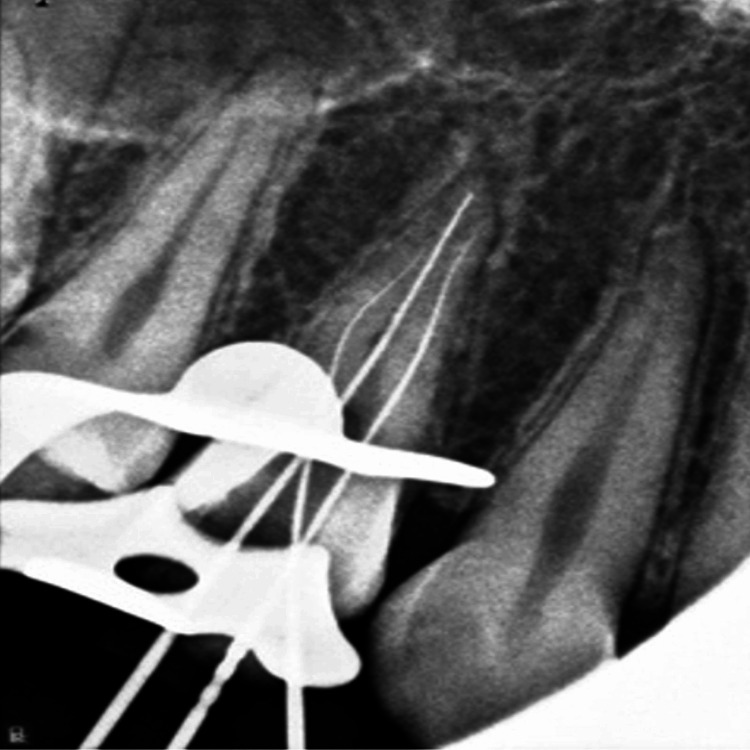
Radiograph of working length
